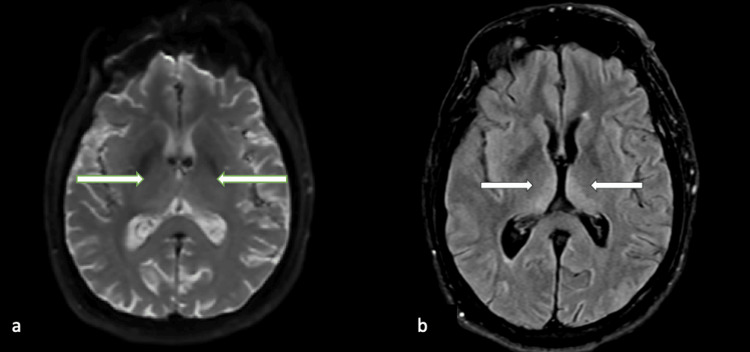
Brain MRI 72 hours post-cardiac arrest a) Diffusion-weighted imaging brain MRI showing no diffusion restriction in bilateral thalami (see arrows). b) Symmetric high FLAIR signal in bilateral medial thalami (indicated by arrows). FLAIR: Fluid-attenuated inversion recovery
